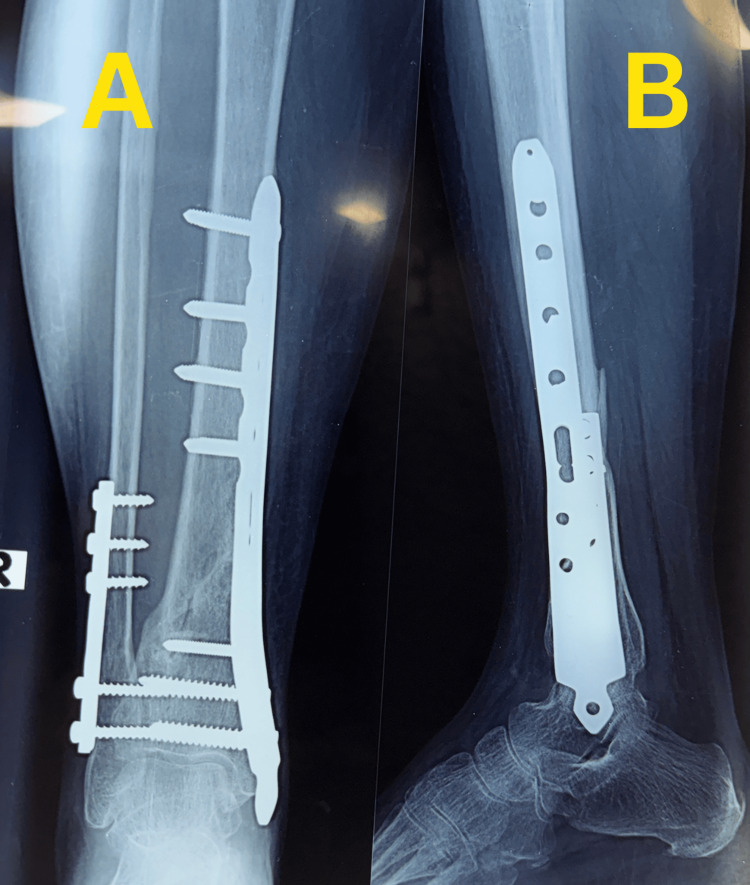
Follow-up radiographs during healing of Case 2. (A) Anteroposterior view demonstrating maintained alignment with visible callus formation at the fracture site. (B) Lateral view showing progressive fracture healing without implant failure or loss of reduction.
